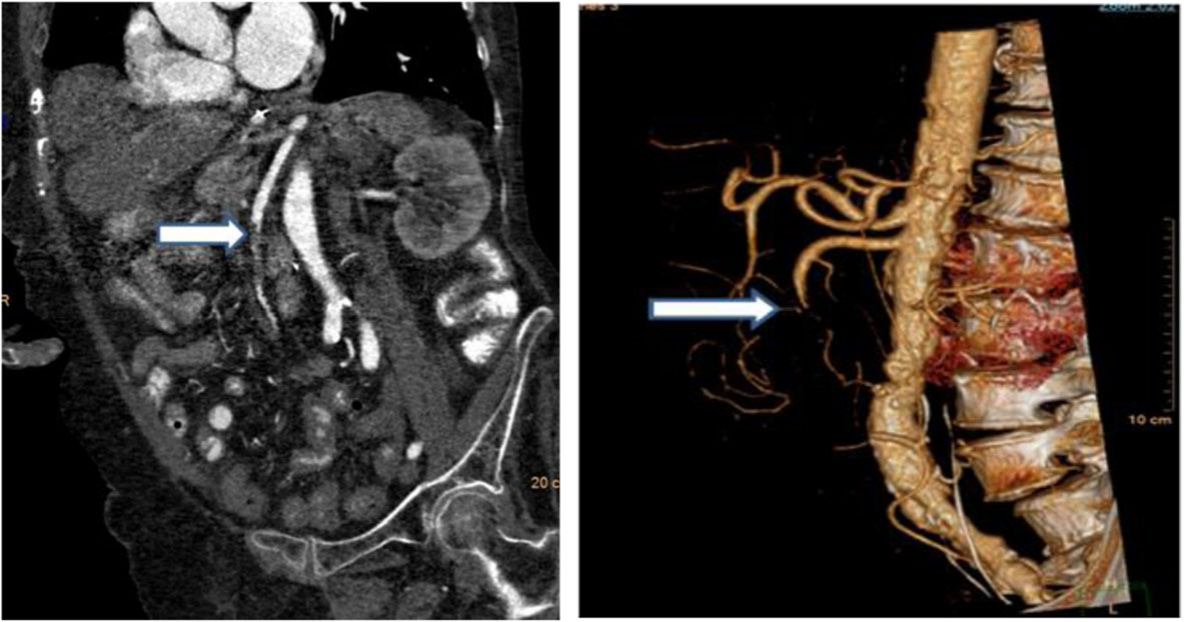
Selected image from a CTA scan of a patient with acute mesenteric ischemia secondary to occluded SMA from an embolic source (arrow). 3D reconstruction is demonstrates mid occlusion of SMA (arrow)
